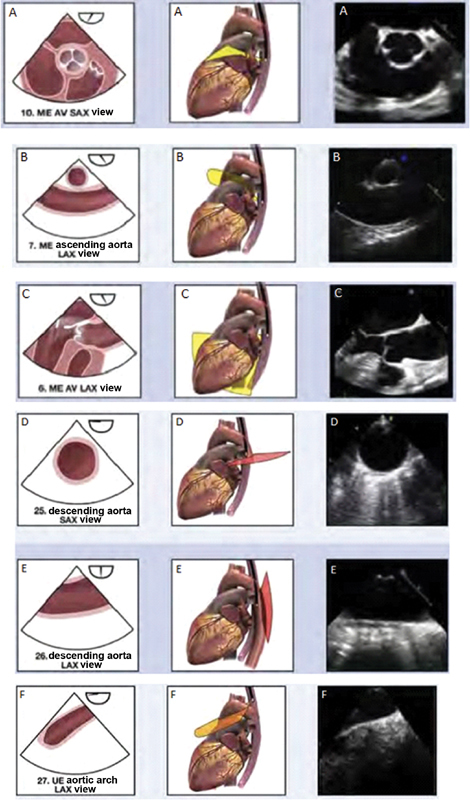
Canonical views on transesophageal echo (TEE): (
